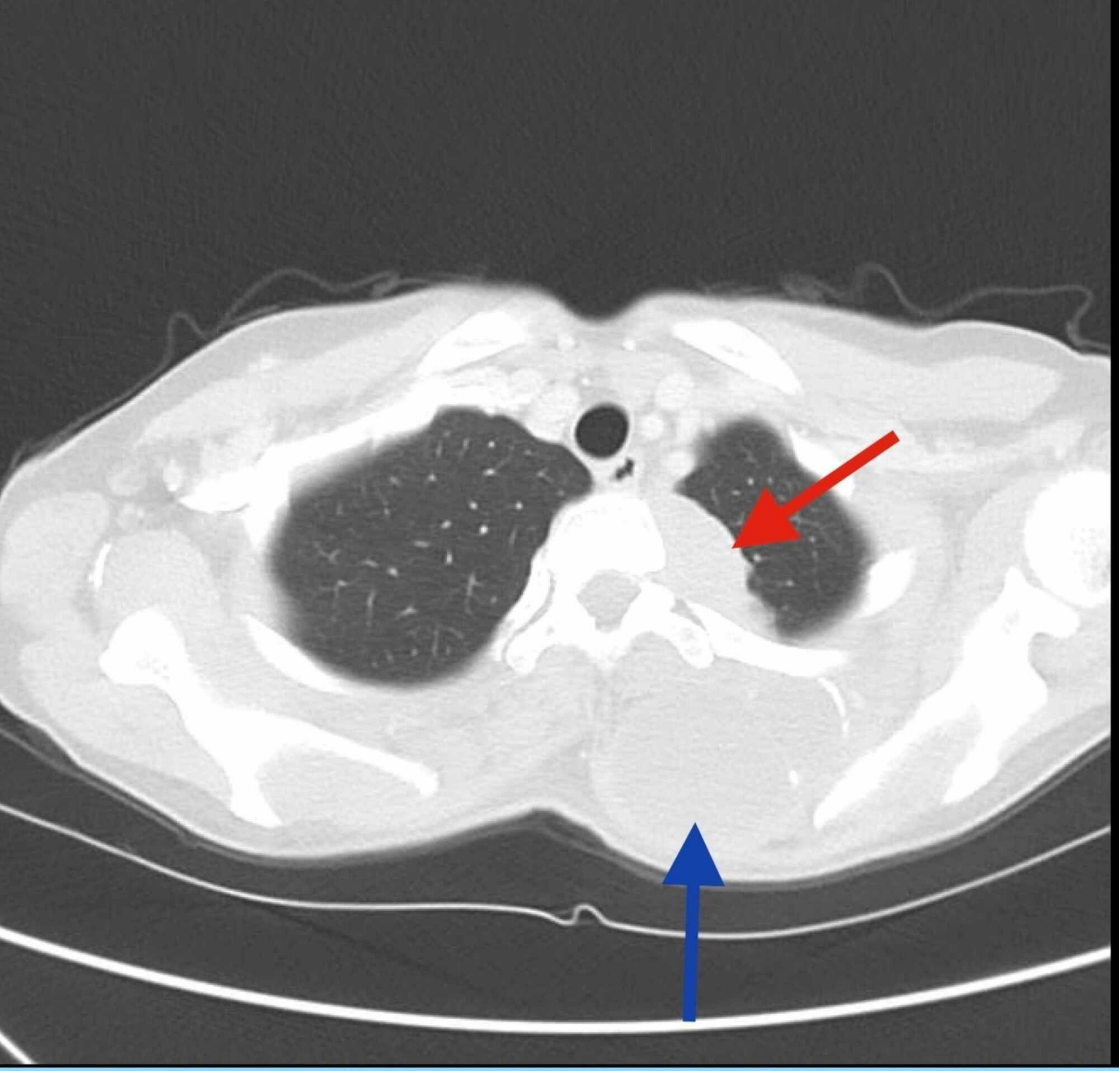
CT scan of axial section showing left pleural nodule (red arrow) and left trapezius muscle mass (blue arrow)
